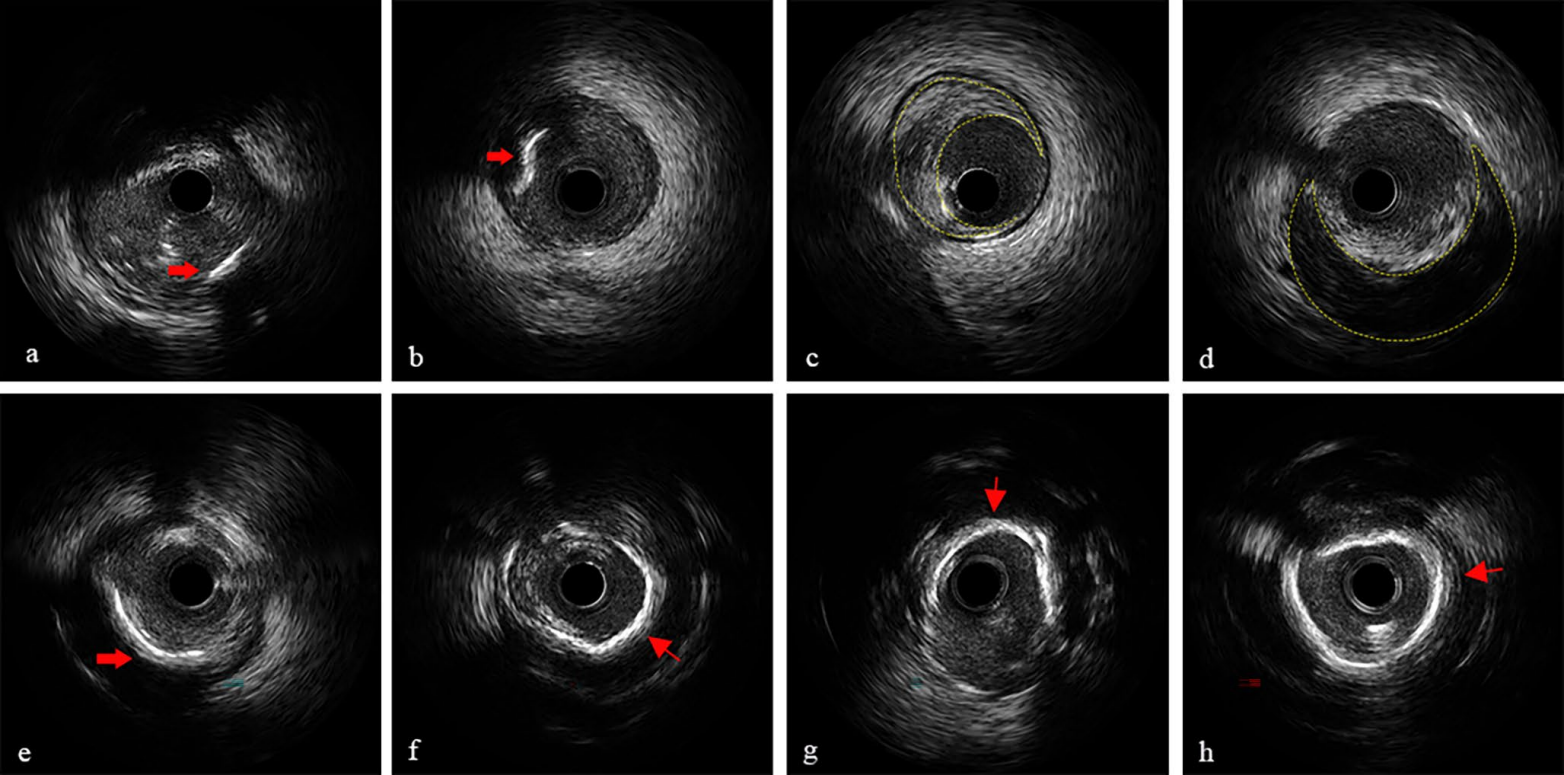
Representative IVUS images (a) Spotty calcification. (b) Calcification nodal. (c) PB > 70%. (d) Lipid-rich plaque. (e) Calcification with arc = 90°. (f) Calcification with arc = 180°. (g) Calcification with arc = 270°. (h) Annular calcification IVUS, intravascular ultrasound; PB, plaque burden
